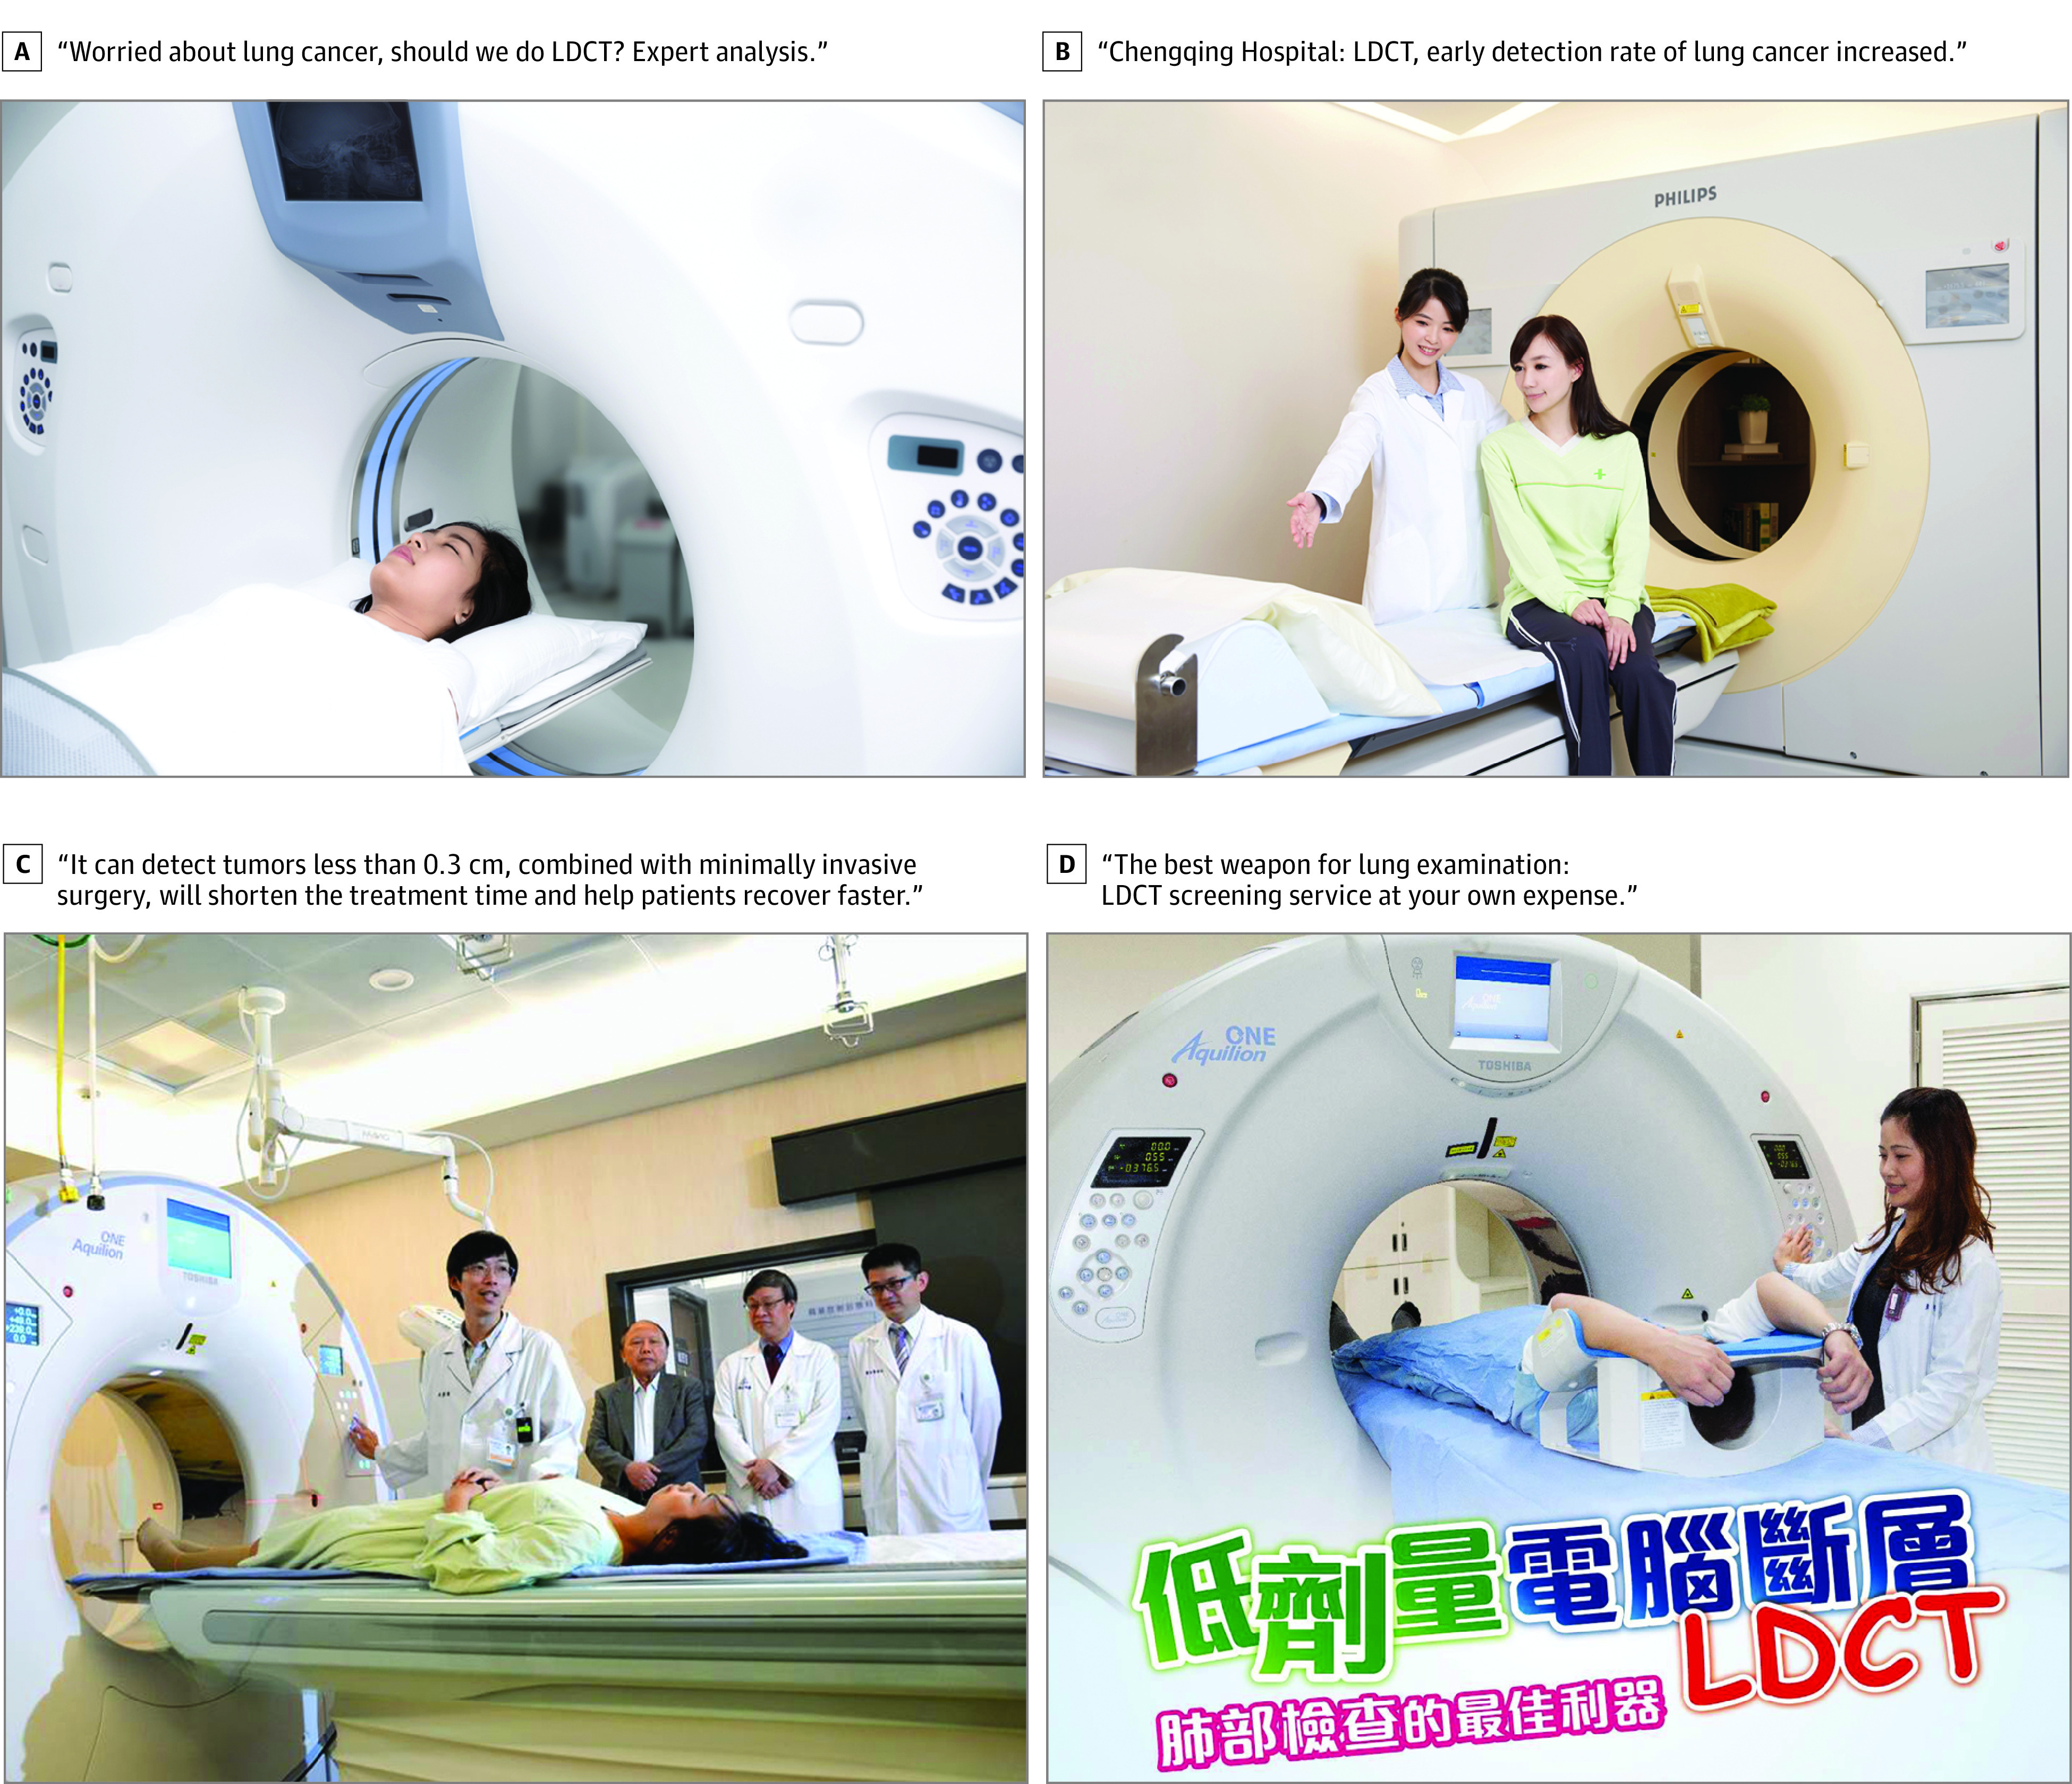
Taiwanese Lung Cancer Screening Promotions Featuring Young Women LDCT indicates low-dose computed tomography. A, Reprinted with permission from Mr Suwannaphoom and copyright holder.^19^ B, Reprinted with permission from the *InfoTimes*.^20^ C, Reprinted with permission from Ms Yao and copyright holder.^21^ D, Reprinted with permission from Department of Medical Imaging and Intervention Chang Gueng Memorial Hospital, Taiwan.^22^
